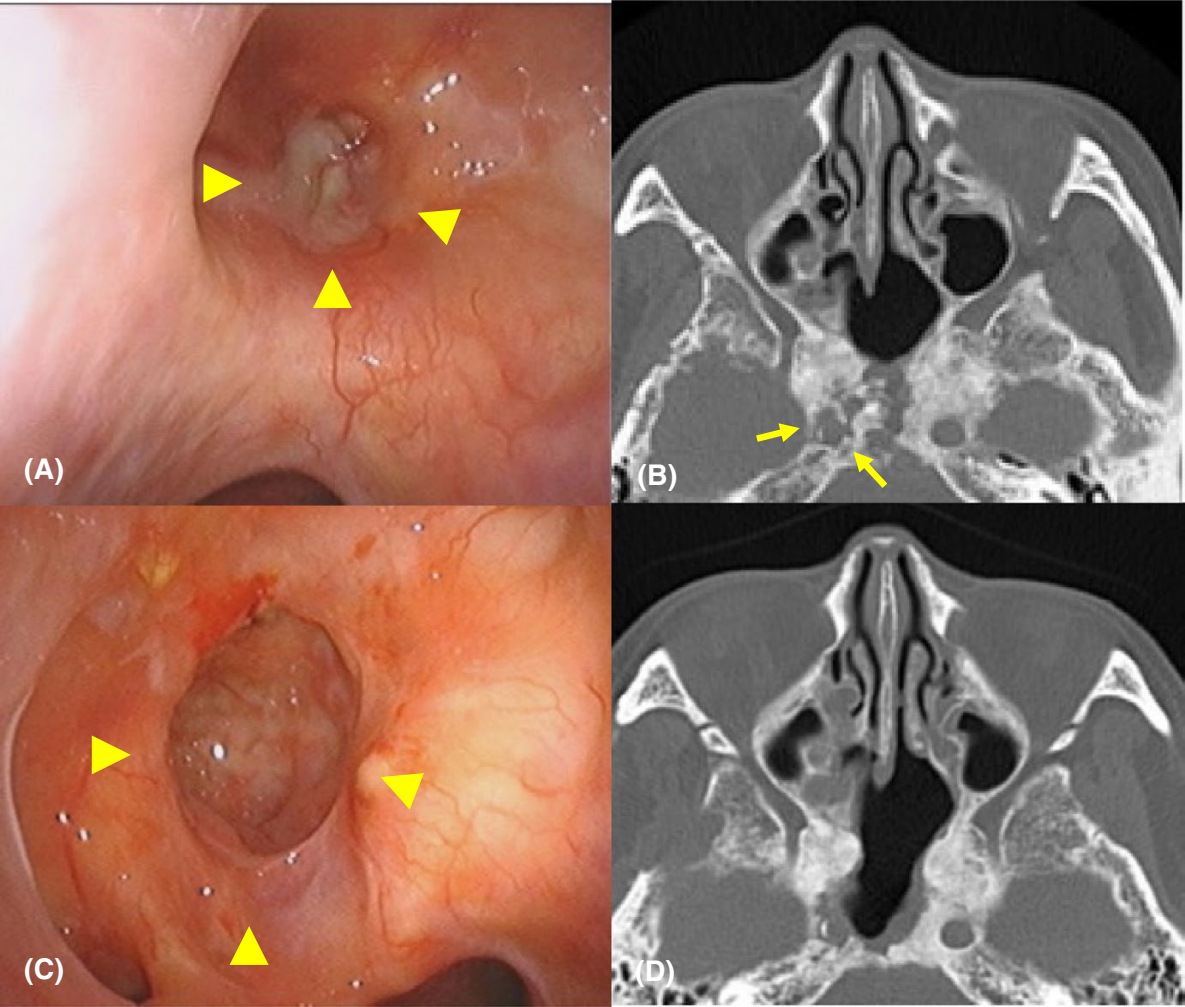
A. Preoperative nasal endoscopic finding indicating the discharge of pus from the infected sphenoid sinus, including osteonecrosis. Arrowheads indicate the sphenoid sinus. B. Preoperative axial computed tomography (CT) showing osteonecrosis of the sphenoid sinus, with damage to the right internal carotid canal. Arrows indicate the right internal carotid canal. C. Postoperative nasal endoscopic findings 15 months after surgery indicating the reconstructed sphenoid sinus with removal of necrotic tissue. Arrowheads indicate the reconstructed sphenoid sinus. D. Postoperative axial CT 15 months after surgery showing the reconstructed sphenoid sinus and skull base
